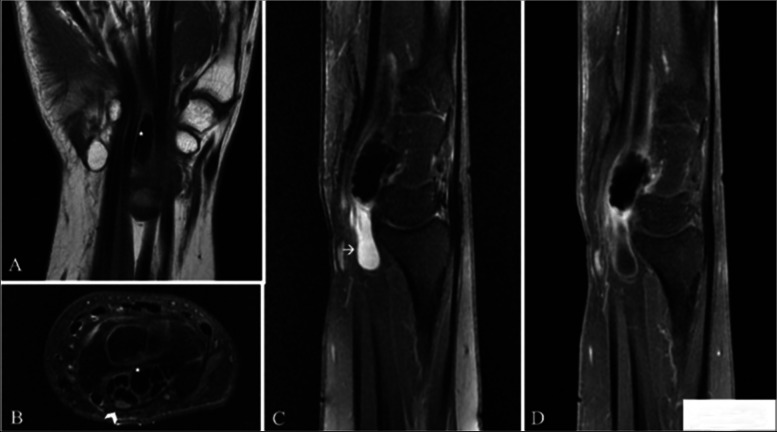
Magnetic resonance imaging of the patient’s right wrist, showing a solitary oval calcification (*), located in the carpal tunnel centrally between the flexor tendons of the wrist, at the lunatum-capitatum junction, without surrounding adherence, having low signal intensity, showing no enhancement. It is surrounded by a reactive fluid collection (arrow), and subtle tenosynovitis of the flexor’s tendon sheaths, with mild compression of the median nerve (arrowhead). A Coronal T1-weighted Fast Spin Echo sequence. B Axial and C Sagittal Proton Density (PD) fat saturated sequences. D Post contrast administration sagittal T1-weighted fat saturated sequence
